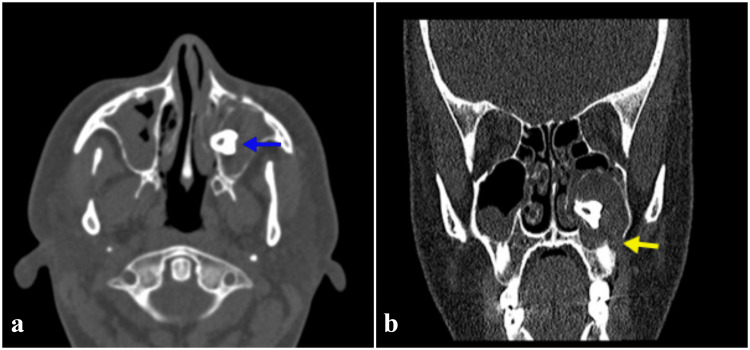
Paranasal Sinus Computed Tomography Images (a) Axial section; the blue arrow indicates the maxillary third molar tooth within the left maxillary sinus. (b) Coronal section; the yellow arrow indicates the defect in the left maxillary sinus inferolateral wall.
